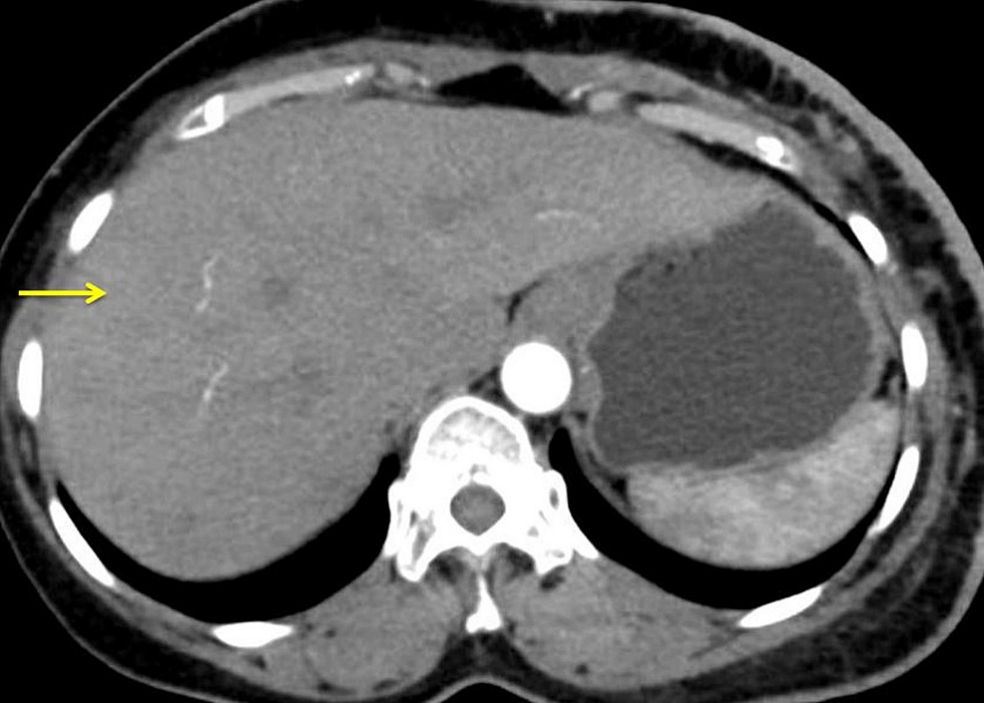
Computed tomography showing normal liver (arrow) without any evidence of distant metastasis.
